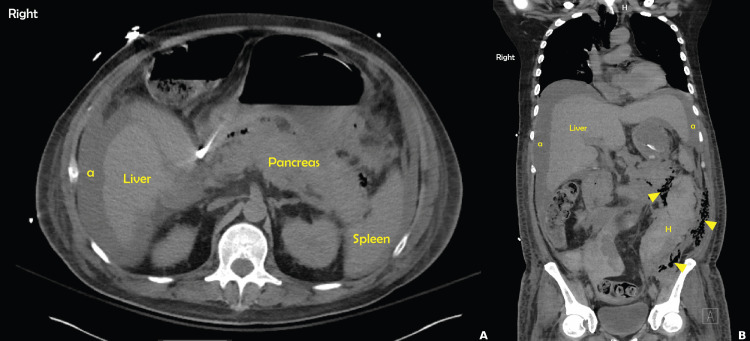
Abdomen and pelvis CT scan A: Axial section demonstrating diffuse interstitial pancreatic edema. There is also the presence of peri-hepatic ascites (a). B: Coronal section showing hemorrhage (H) in the retroperitoneum. There are also large amounts of gas surrounding the hemoperitoneum, which can be suspicious for gas gangrene (arrows). There is also a presence of abdominal ascites (a).
